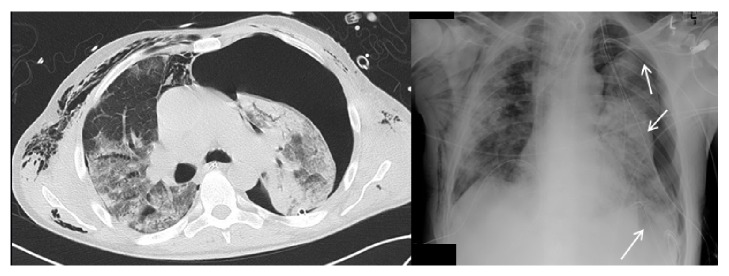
CT-scan (left image) and chest X-ray (right image) on day 16 before endobronchial valve placement: large pneumothorax despite three chest drainages on the left (white arrows).
